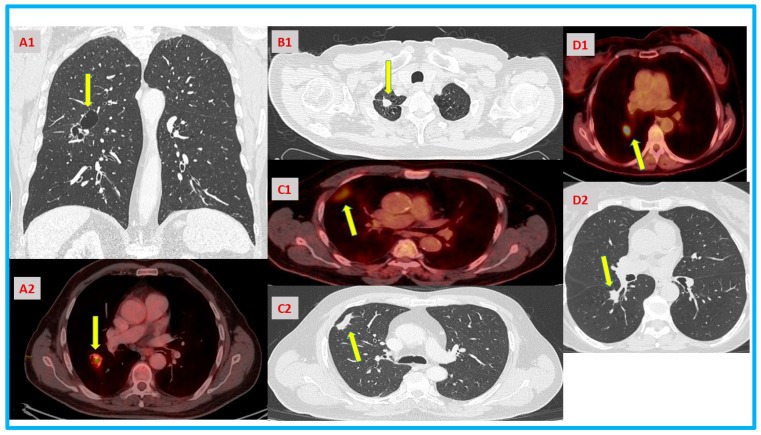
(A–D) Computed tomography and PET-CT scans of the presented patients. (A1) The arrow marks the suspicious cavitary lesion in the major fissure extending from the RLL to the RUL. (A2) The arrow marks the central hypermetabolic FDG avid nodule in the RLL. (B1) The arrow marks the apical mass in the vicinity of the emphysematous lung. (C1) The arrow marks the peripheral consolidation (on biopsy: chronic inflammation). (C2) The arrow marks the peripheral hypermetabolic FDG avid consolidation (on biopsy: chronic inflammation). (D1) The arrow marks a central hypermetabolic FDG avid nodule near the segmental pulmonary artery (biopsy was negative for tumor). (D2) The arrow marks central nodule (biopsy was negative for tumor).
